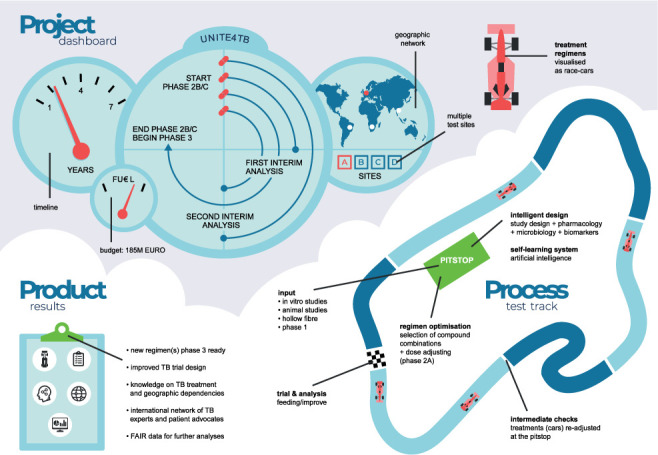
The UNITE4TB racetrack concept for the development of new drugs and regimens: the individual compounds are represented as essential car parts (e.g., tyres, engine, etc.). When ready, they enter the pitstop, where they are prepared to be incorporated into a car, or new treatment regimen. Potential regimens are launched onto the racetrack (the CT platform) to participate in the race (to select the best car or treatment regimen). FAIR = Findability, Accessibility, Interoperability, Reusability; CT =clinical trial.
